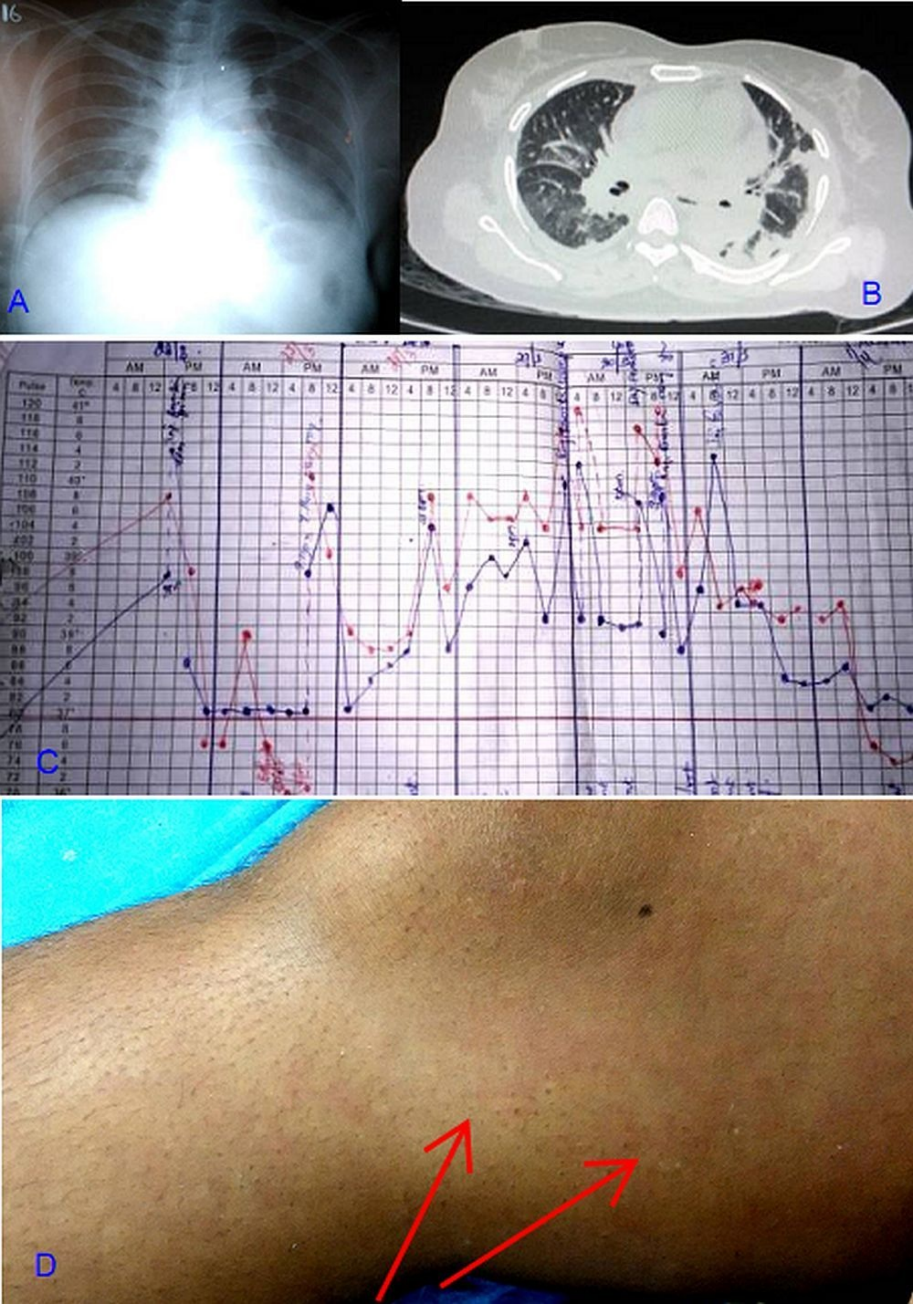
The patient's imagings, fever chart, and rash a. Chest radiograph showed cardiomegaly and left costophrenic angle blunting. b. Chest computed tomography revealed bilateral mild pleural effusion with consolidation along the medial basal segment of the left lower lobe and medial segment of the right middle lobe. c. Temperature chart shows fever crashing on day 2 (first day in ICU) and high spiking temperature for the next four days. d. Right thigh shows salmon-colored rash, which appeared on day 4.
